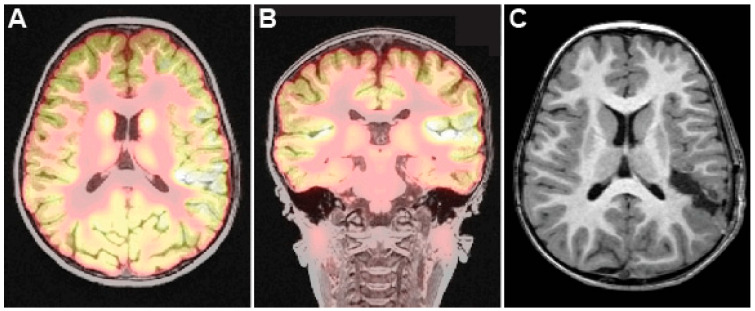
Pre- and postoperative imaging for Case 2. Axial (A) and coronal (B) preoperative PET-MRI demonstrating hypermetabolism in left posterior peri-sylvian region. (C) Postoperative axial T1-weighted MRI demonstrating resection of posterior insula and overlying opercula.
